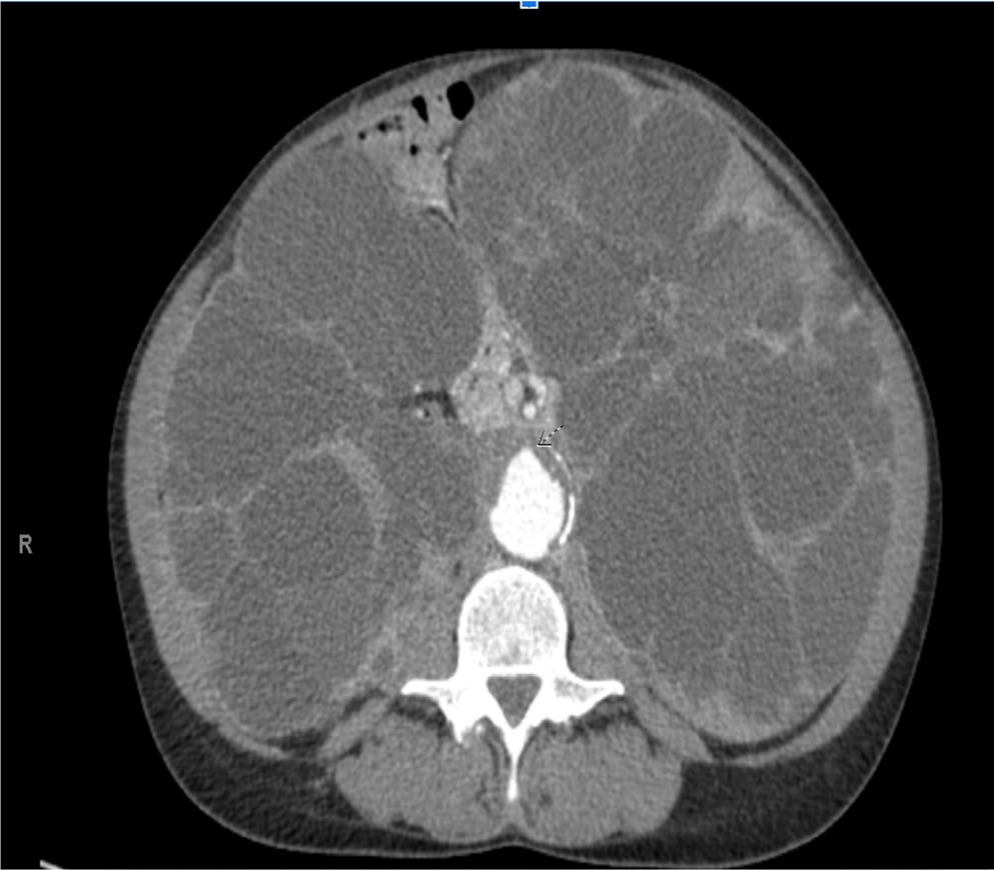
CT scan of the abdomen, axial view showing bilateral enlarged kidneys, more on the left, causing compression of theIVC.
